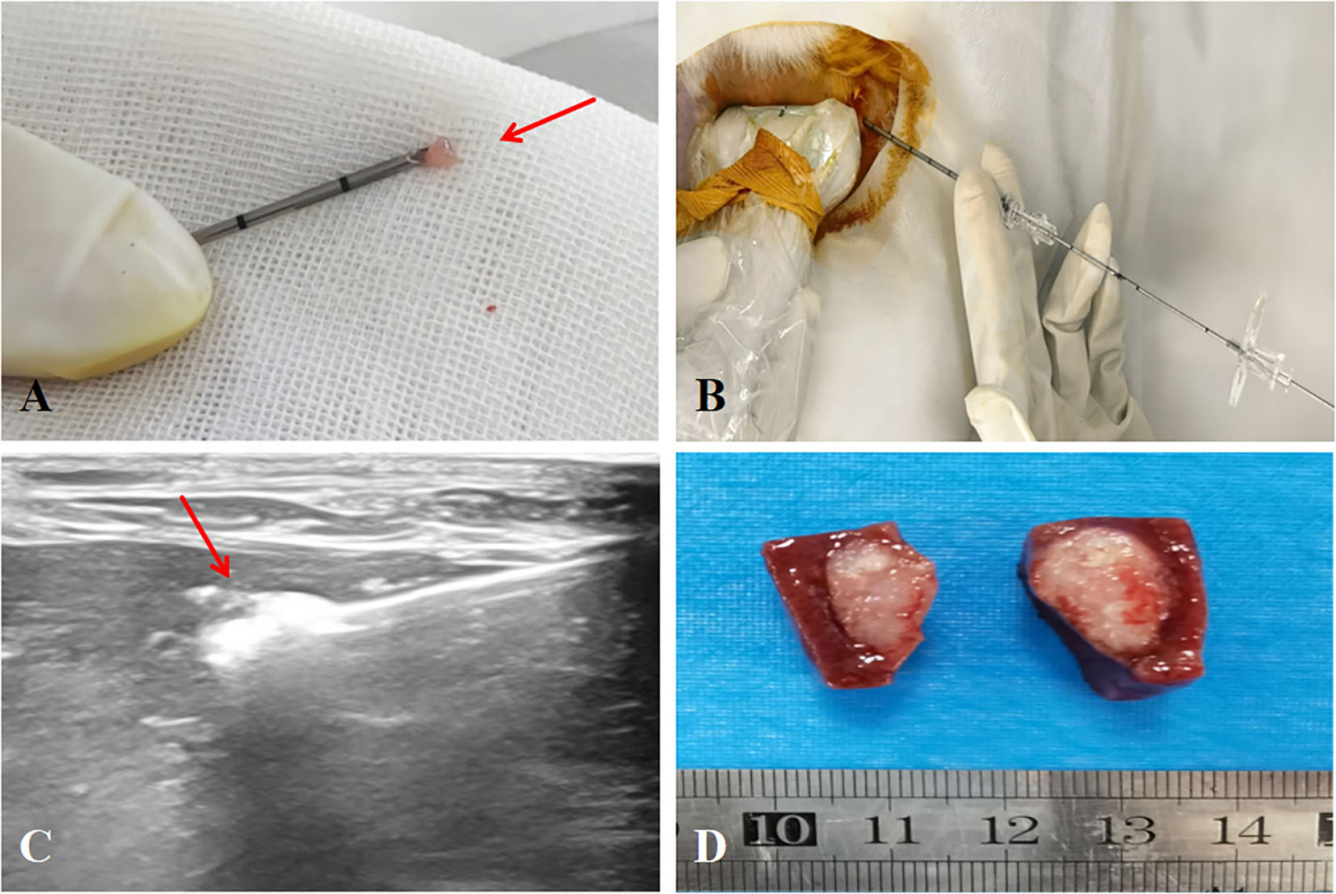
Ultrasound-guided implantation modeling of VX2 tumors in rabbit liver. (A) Insert the tumor pieces of 1 - 2mm³ into the needle sheath from the tip(→). (B) The needle was inserted into the target area under ultrasound guidance. (C) Use the matching needle core to push the tumor pieces in the needle sheath into the liver(→). (D) Liver VX2 tumor model.
